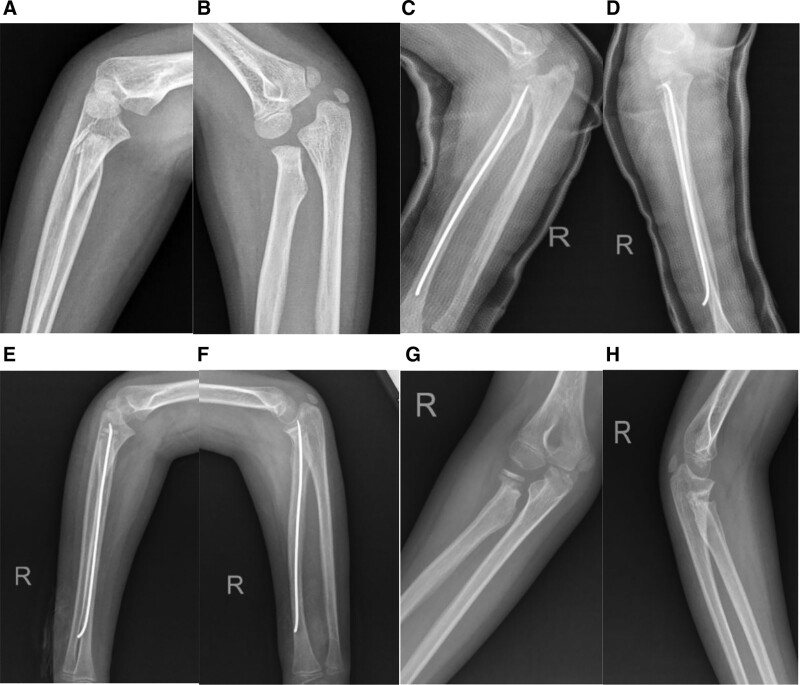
Case of ESIN shifting and joint protrusion: (A and B) initial AP and lateral radiographs demonstrating a Judet type IV radial neck fracture (RNF); (C and D) elbow radiographs taken on the first postoperative day; (E and F) AP and lateral radiographs at a 45-day postoperative follow-up, showing ESIN shifting and intrusion into the joint; (G and H) radiographs taken at a 6-month postoperative follow-up, revealing malunion of the radial neck.
